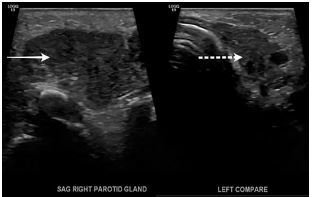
Parotid point-of-care ultrasound: swollen right-side parotid gland (solid white arrow) compared to the left-side parotid gland (dotted white arrow).
